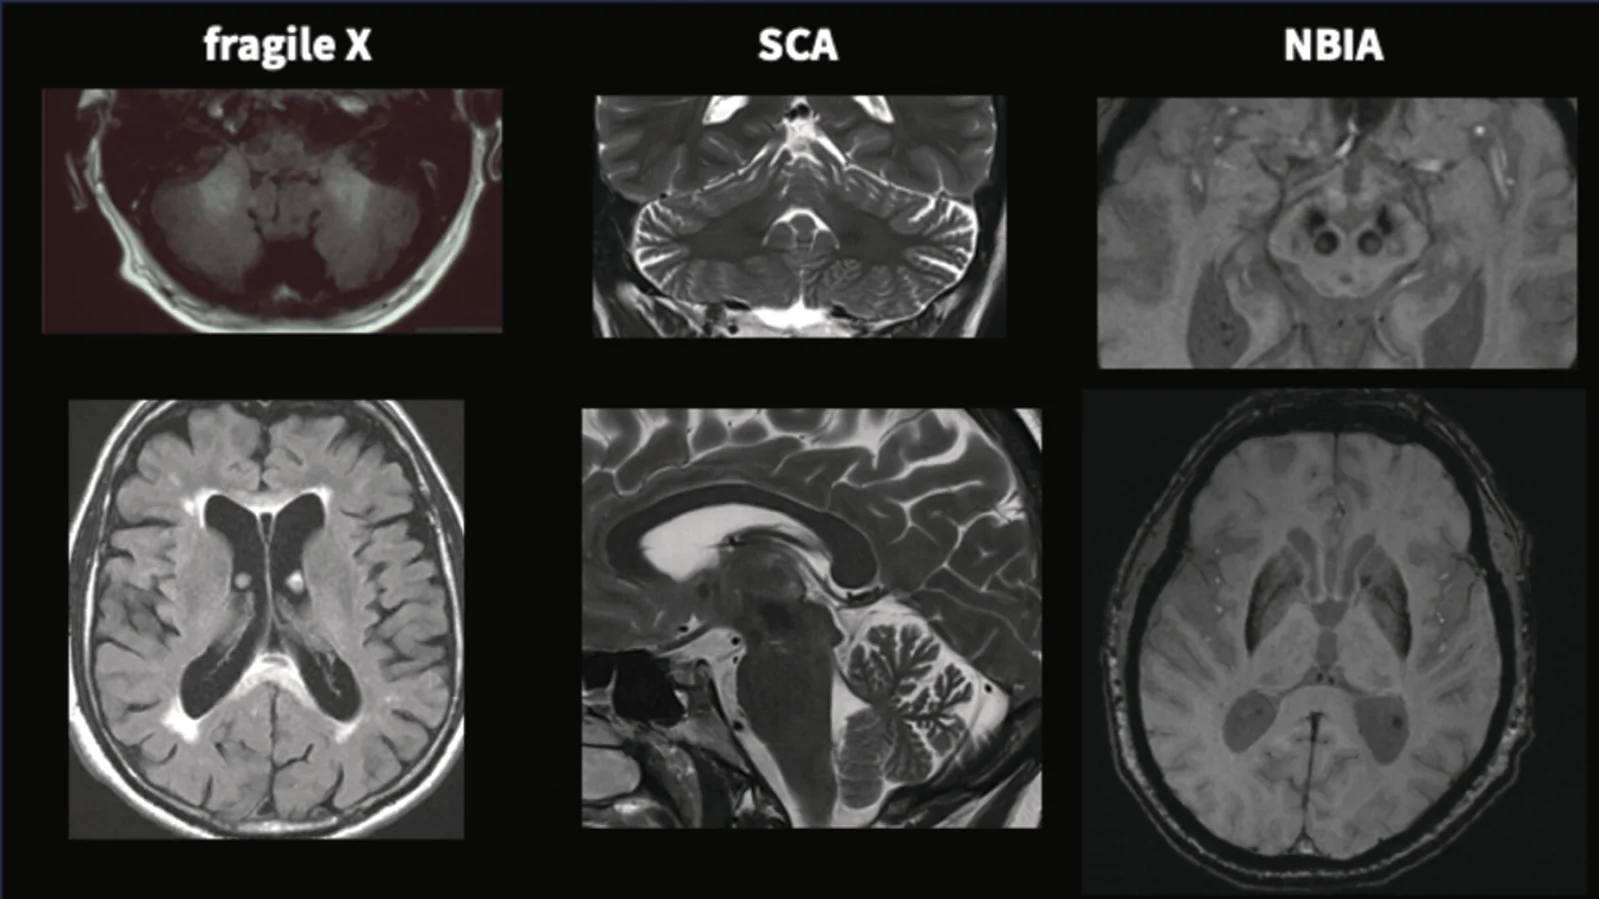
MRI findings in genetic and neurodegenerative tremor syndromes. Structural MRI can reveal characteristic abnormalities in tremor syndromes with additional neurological features. Fragile X–associated tremor/ataxia syndrome (FXTAS) is associated with cerebral and cerebellar atrophy and T2/FLAIR hyperintensities of the splenium and middle cerebellar peduncles. Spinocerebellar ataxias (SCA) show cerebellar atrophy. Neurodegeneration with brain iron accumulation (NBIA) shows abnormal iron deposition in the basal ganglia, most prominently the globus pallidus and substantia nigra, with the “eye-of-the-tiger” sign being characteristic of pantothenate kinase-associated neurodegeneration.
